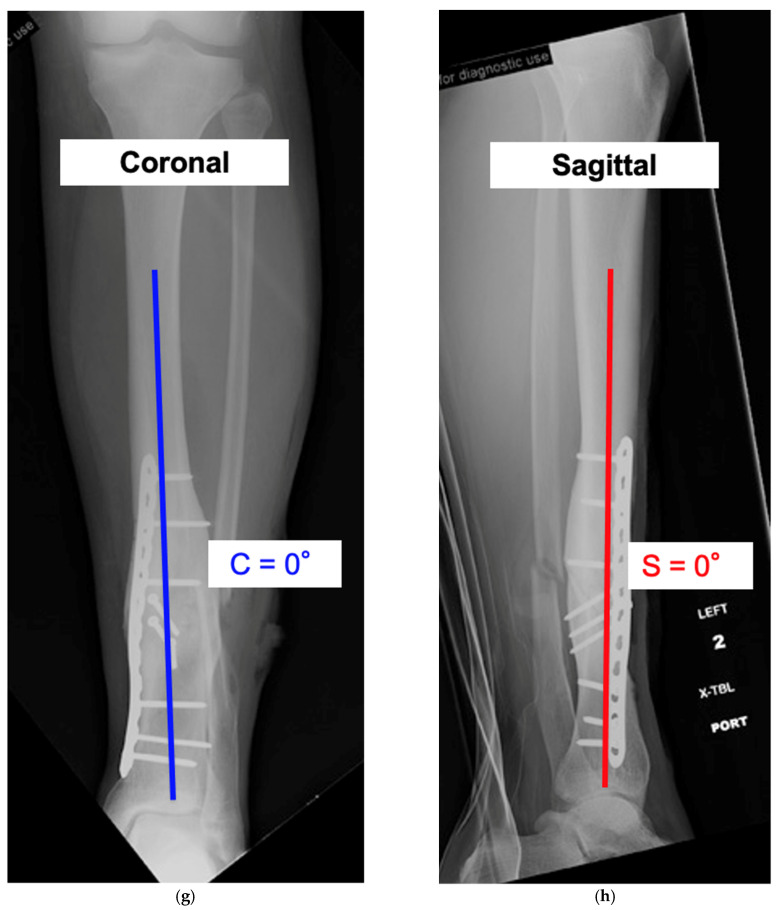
See Case 2 description for details of a tibial malunion. (a–h) calculate the variables θ (Osteotomy Angle) and φ (Starting Point Angle) to derive the mathematically directed osteotomy. Intraoperative and postoperative radiographs reveal successful correction of the deformity and osteotomy union.
